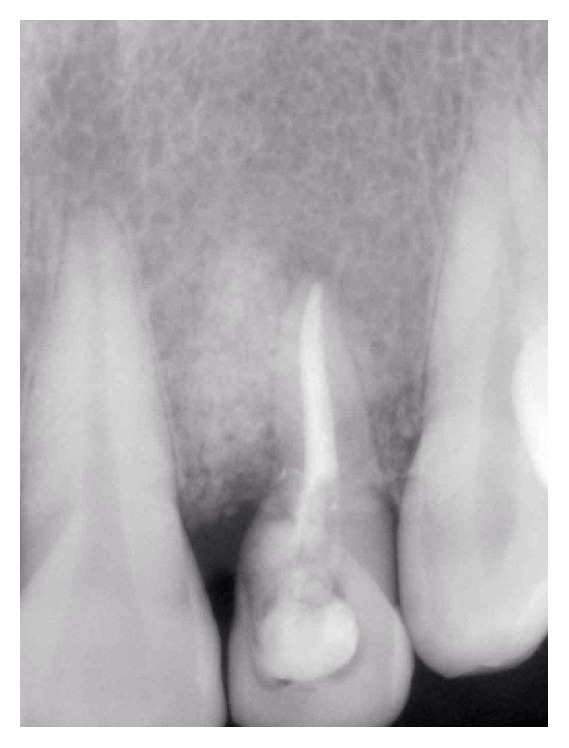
12 months' follow-up radiograph indicated disappearance of the radiolucency around the tooth #10 due to bone grafting and simultaneous bone regeneration.
